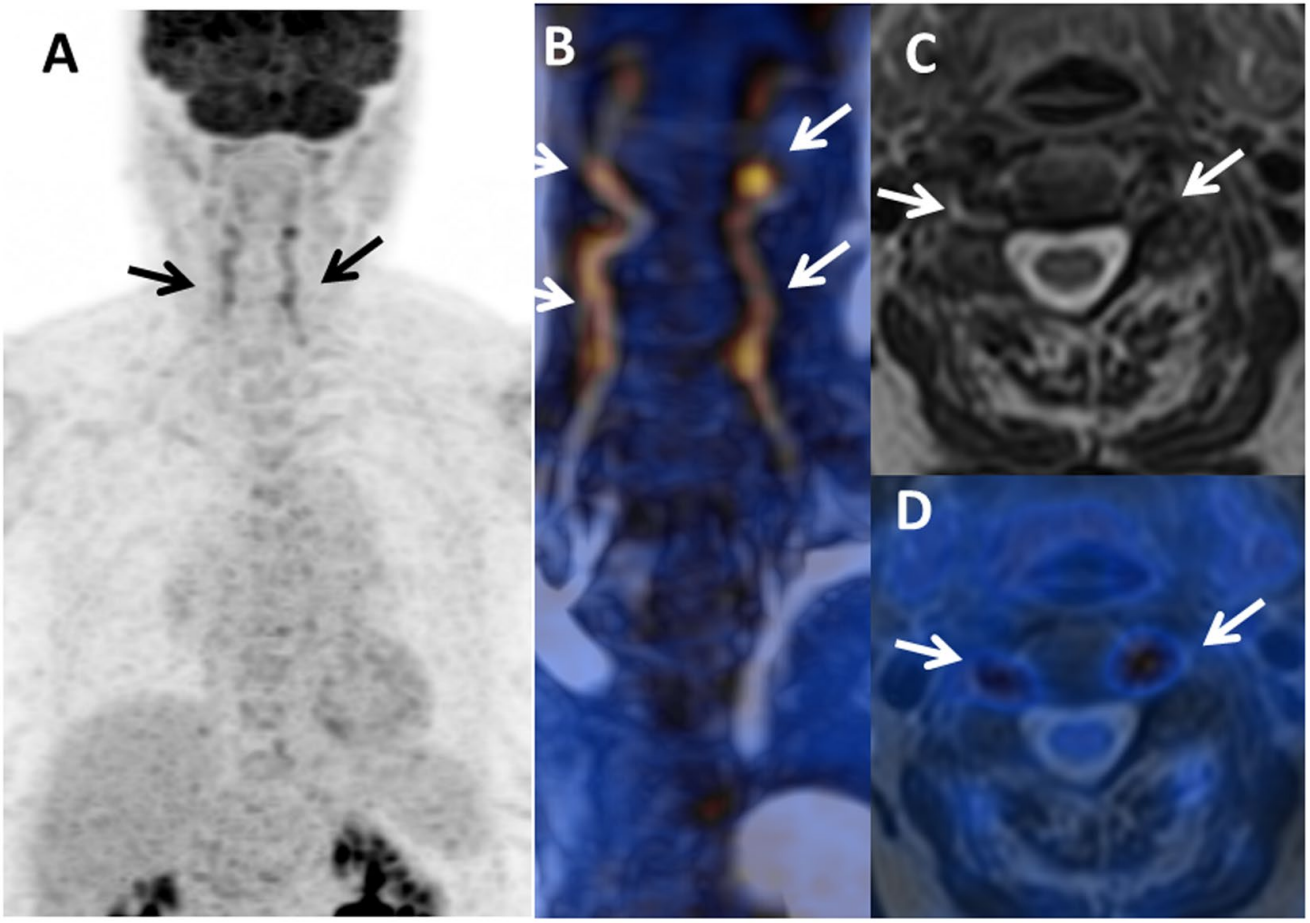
PET/MRI used for the initial diagnosis of giant cell arteritis (GCA) in a female with temporal headaches and acute-phase reactants without any vascular signs or arthralgia. PET/MRI showed an inflammatory pattern with clear uptake (>liver uptake, grade 3) in vertebral arteries ((A) Maximum intensity projection, and (B) fusion MR angiography/PET; arrows) associated with arterial wall thickening on: (C) MR axial T2-weighted image and (D) T2-weighted/PET fusion.
